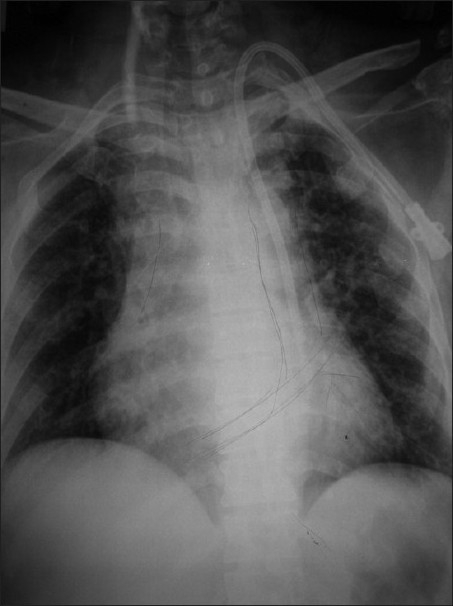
Chest radiograph showing the hemodialysis catheter passing through a persistent left superior vena cava
